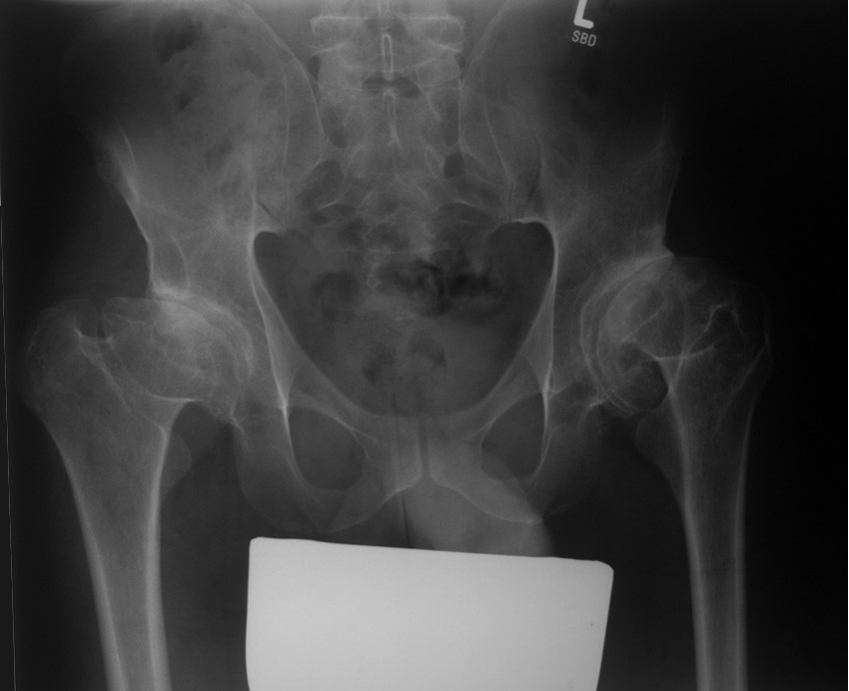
X-ray of the pelvis (anterior posterior view) demonstrating deformity of the femoral heads and osteoarthritis of the hip joints.
